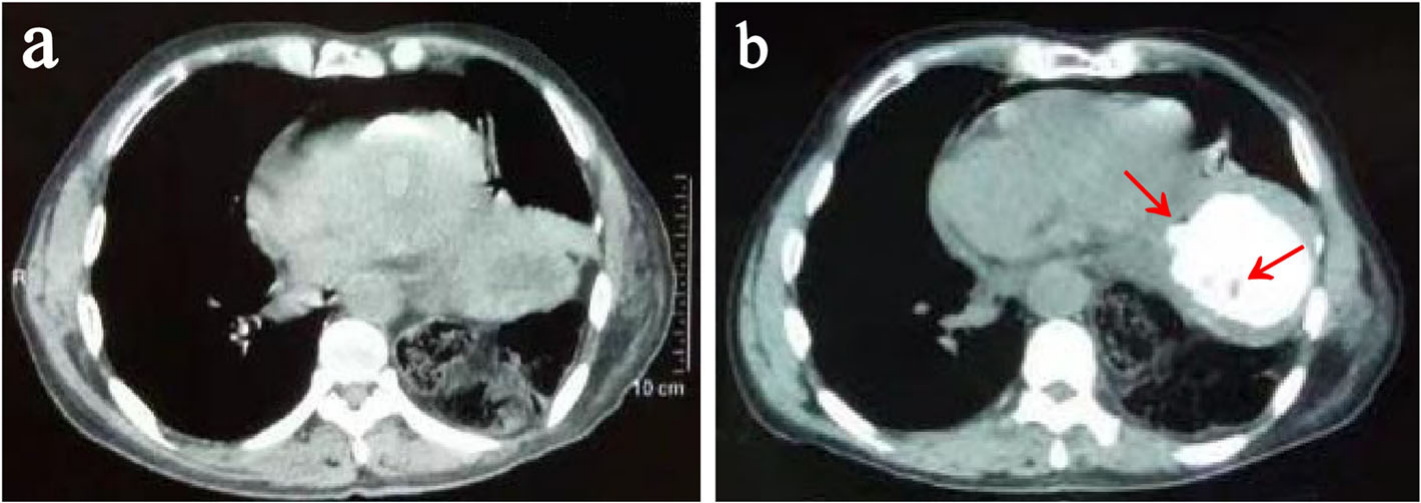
Preoperative CT. a CT revealed the diaphragmatic hernias and air collection within the pericardial space. b There was no contrast agent leak into the pericardial space while a gastric wall ulcer and an unspecific low density in the thoracic gastric cavity were noticed
